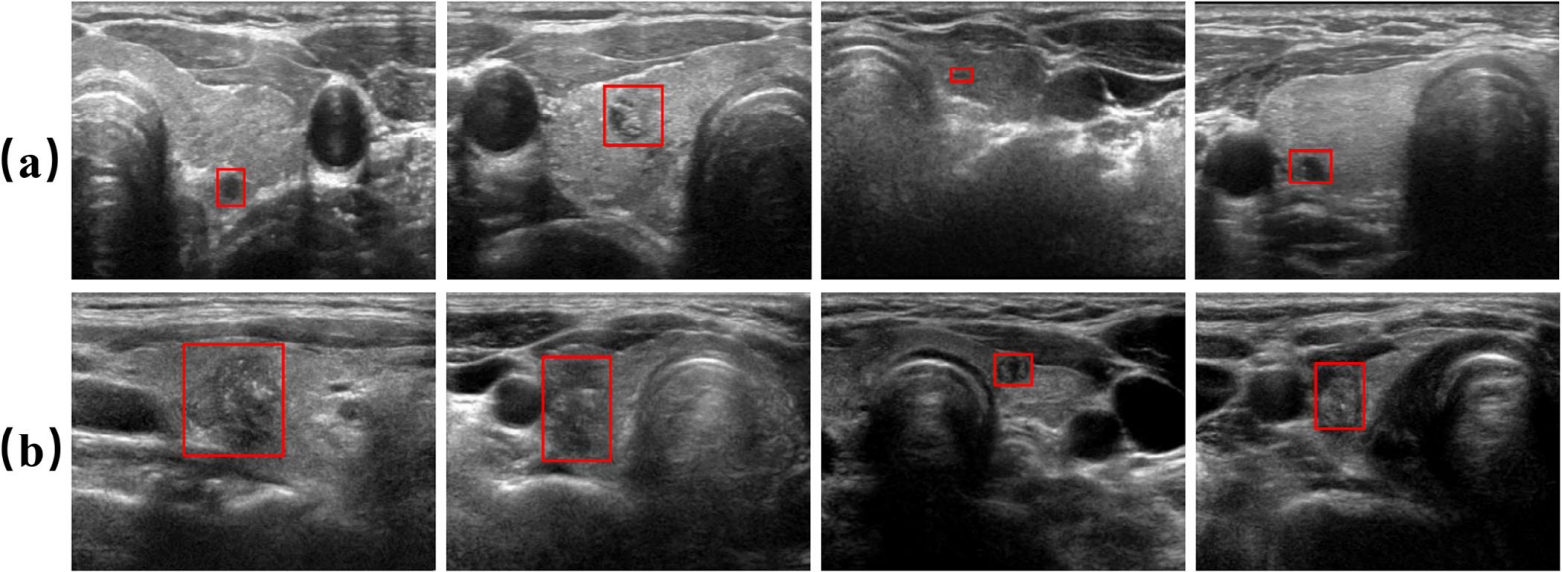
Examples of thyroid nodules in ultrasound images. (a) benign, (b) malignant. The red box marks the location and shape of the nodule.
